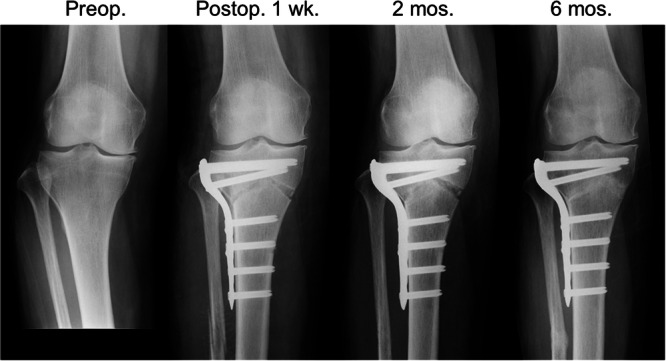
Serial radiographs after inverted V‐shaped high tibial osteotomy (iVHTO). Anteroposterior knee radiographs obtained preoperatively and at 1 week, 2 months, and 6 months postoperatively demonstrate maintenance of correction and progressive healing at the osteotomy site, with complete bone union achieved by 6 months.
